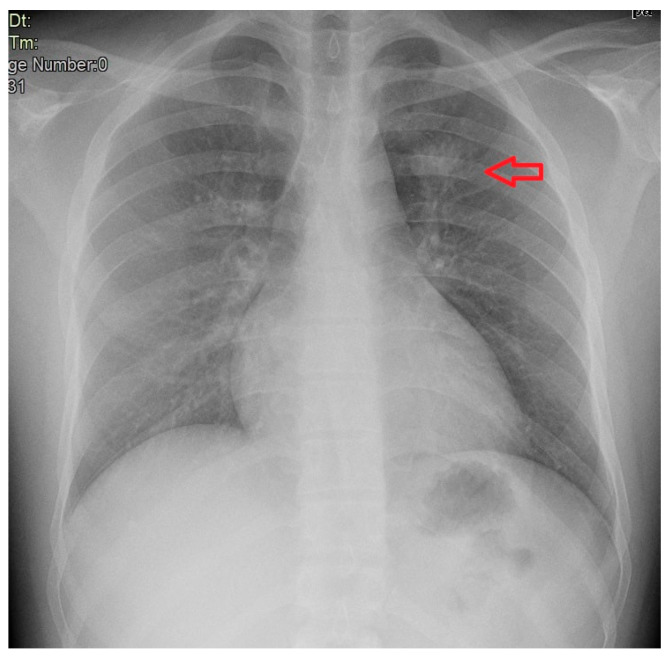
Chest X-ray of case n. 2.
